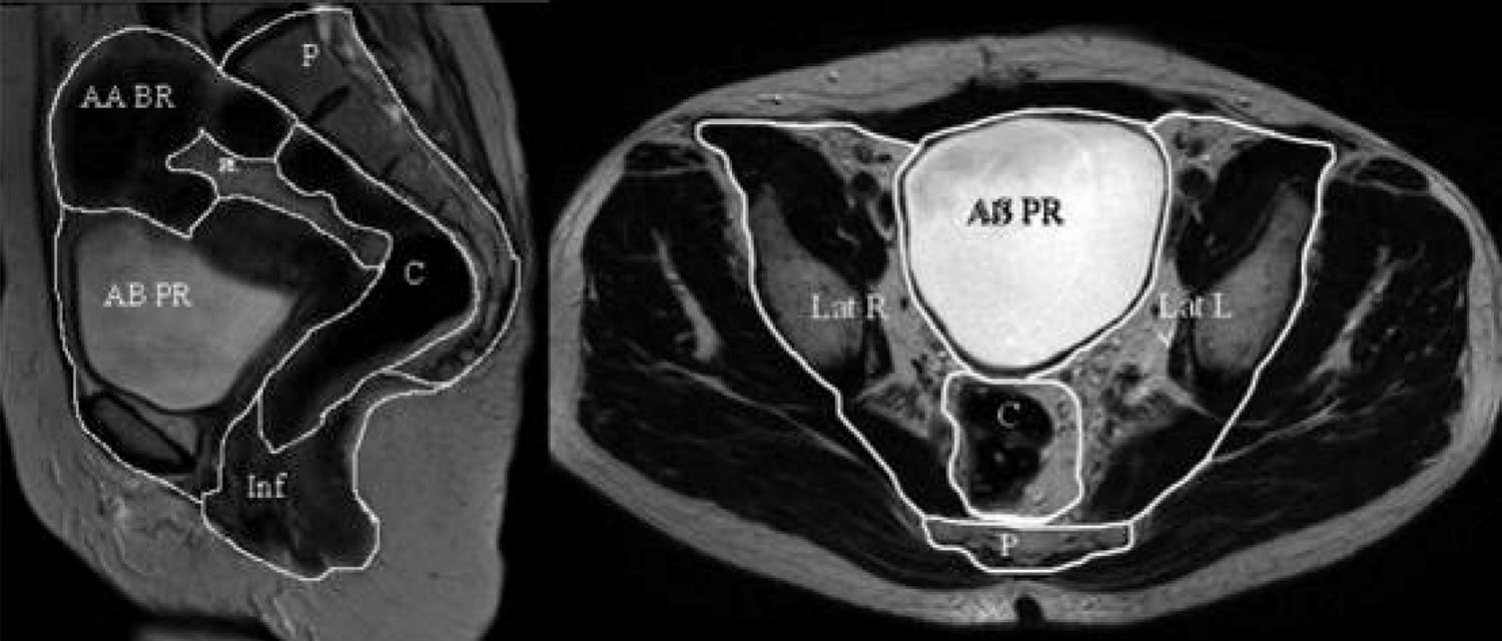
Sagittal and axial T2-weighted magnetic resonance imaging (MRI) images showing the seven intrapelvic compartments introduced in [1]
